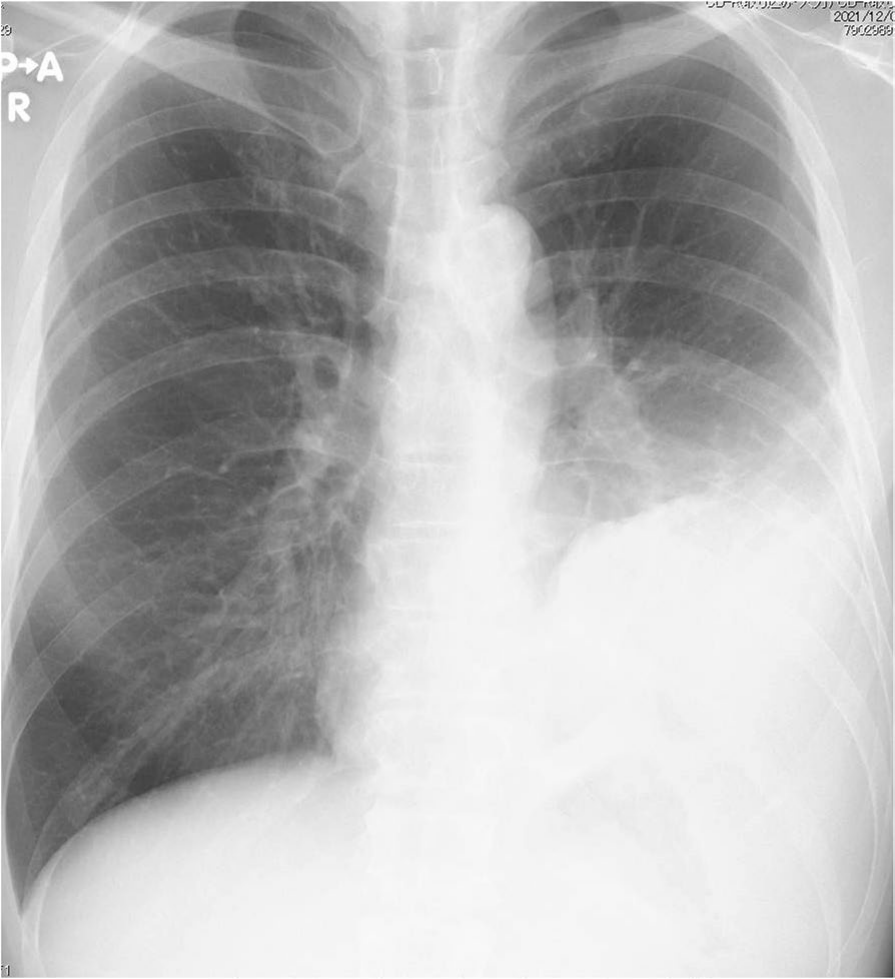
Chest x-ray showing dense opacity pleural effusion in the lower left lung fields
